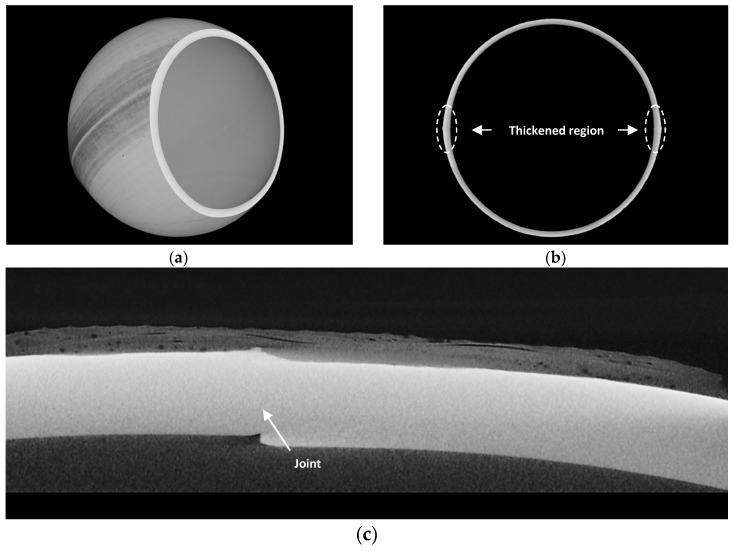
Industrial computed tomography (CT) images of a Si_3_N_4_ floatation sphere. (a,b) CT scanning. (c) Slight misalignment at the joint.
